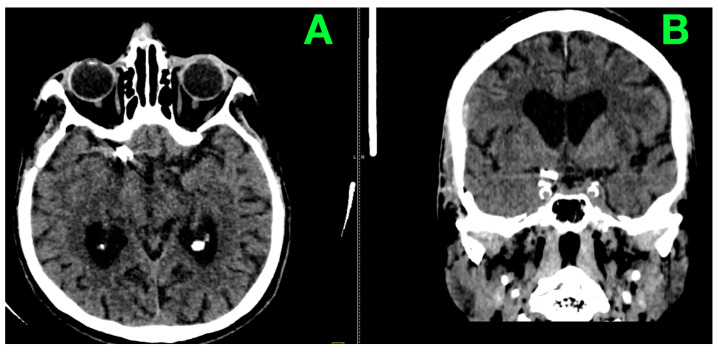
Three-month postoperative non-contrast CT scan. (A): Axial CT scan demonstrates the presence of the right temporofrontal craniotomy flap, with no evidence of complications at the surgical site. The aneurysm clip remains in its correct position at the right carotid cistern, with no associated artifact-related distortions affecting adjacent structures. The basal cisterns and cerebrospinal fluid spaces are clear, and there is no evidence of active or recent hemorrhage. (B): Coronal CT reconstruction highlights marked cerebral atrophy, with compensatory dilatation of the lateral ventricles. The ventricular system demonstrates no obstruction, and the midline structures remain symmetrically aligned. The absence of hemorrhagic changes or ischemic complications confirms the stable postoperative outcome.
